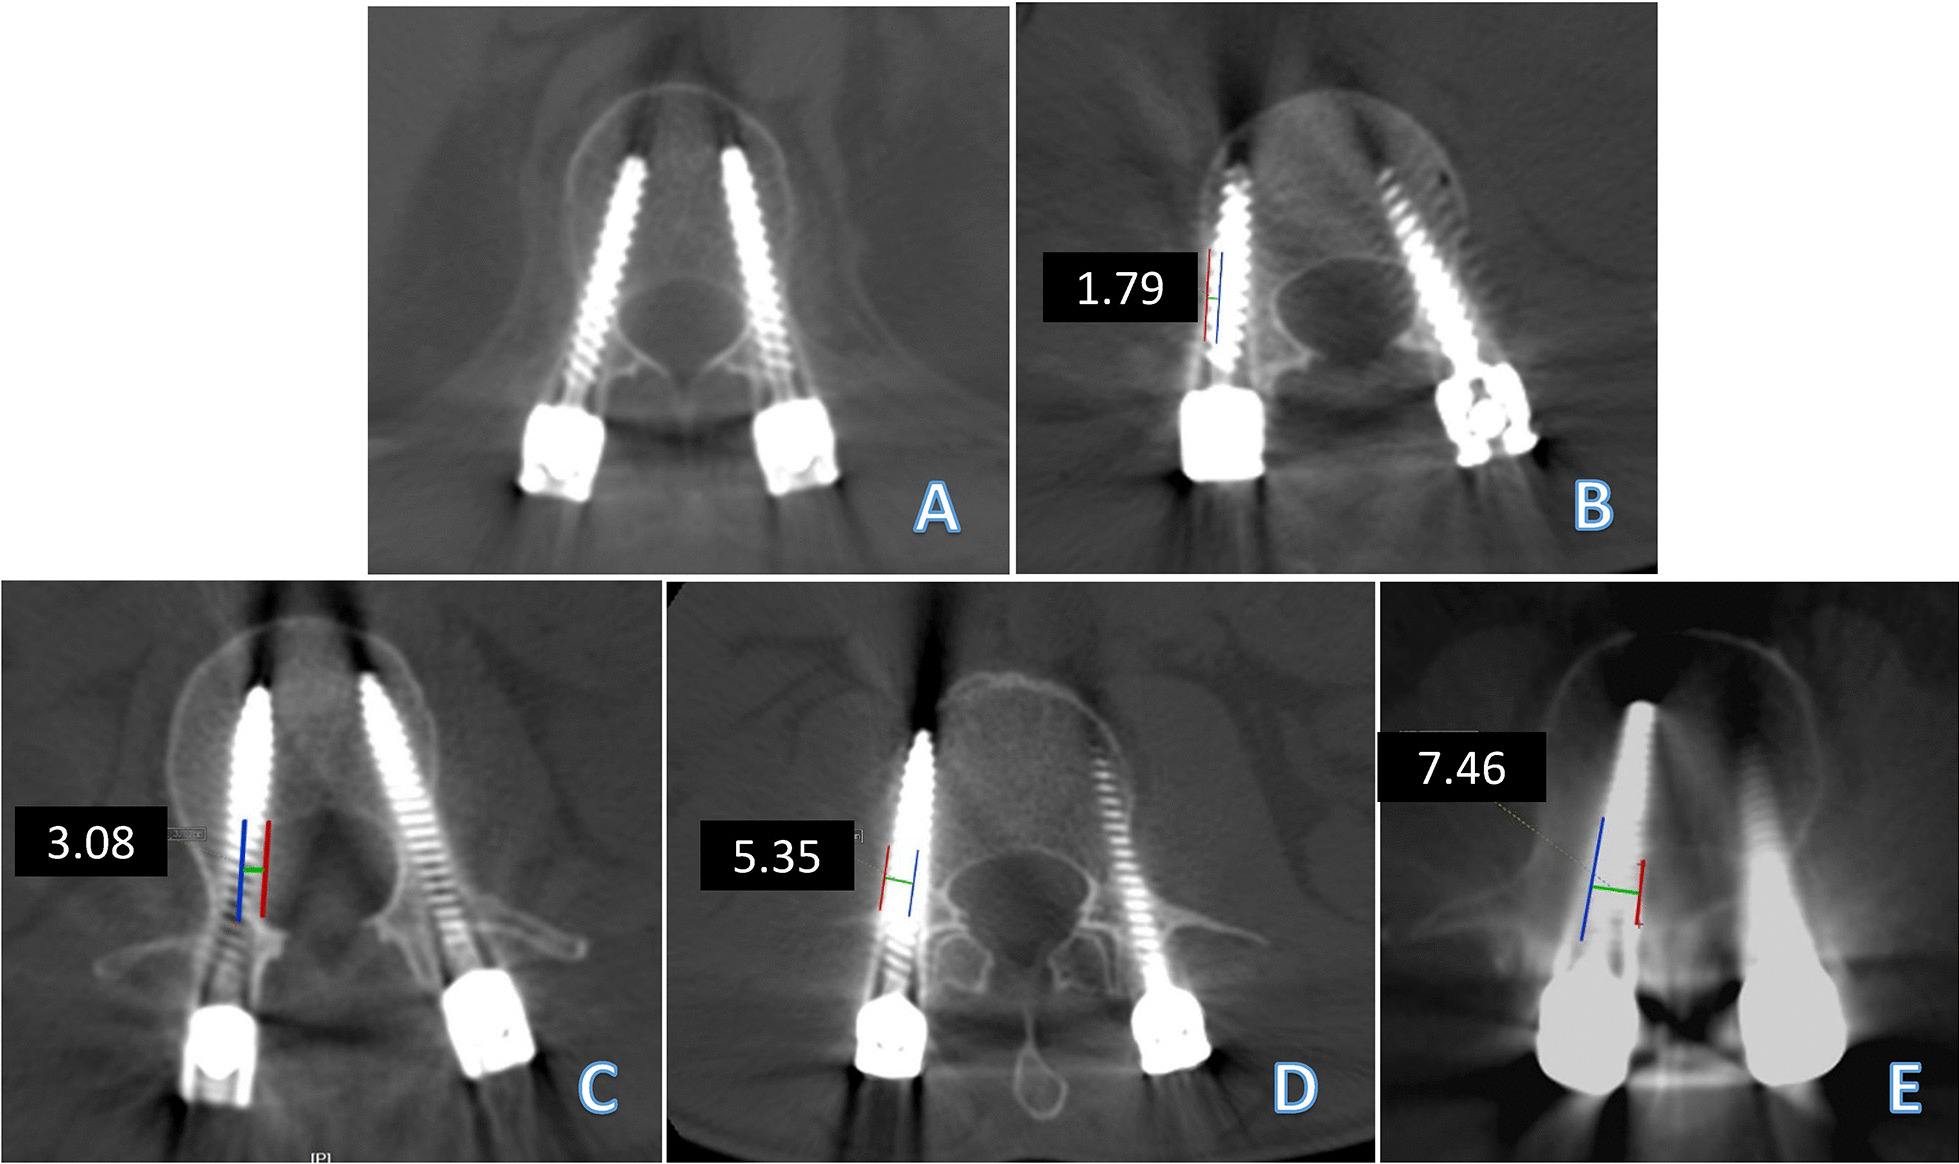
Grading used for pedicle perforation on the axial CT scan and the representative images: A both pedicle screws are completely within the pedicle (Grade A); B Grade B (< 2 mm) perforation of the lateral wall of the right pedicle and Grade A of the left pedicle; C Grade C (2–4 mm) perforation of the medial wall of the left pedicle and Grade A perforation of the right pedicle; D Grade D (> 4 mm) perforation of the lateral wall of both pedicles; E Grade E (> 6 mm) perforation of the medial wall of the right pedicle. The blue lines represent the medial or lateral wall of the vertebral pedicles; the red lines represent the edge of the pedicle screws; and the green lines represent the distance of the perforation
